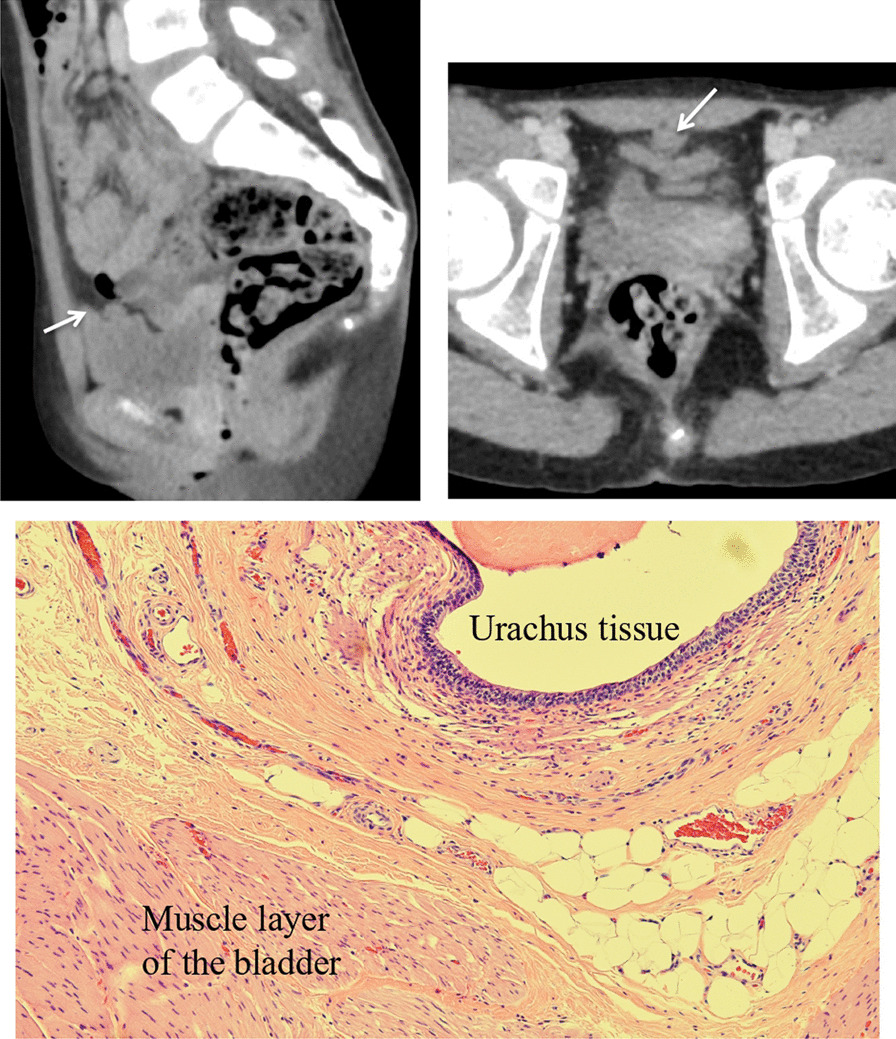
CT showed the urachal remnant at the bladder apex (white arrow). Pathological findings indicated urachus tissue at the bladder apex
